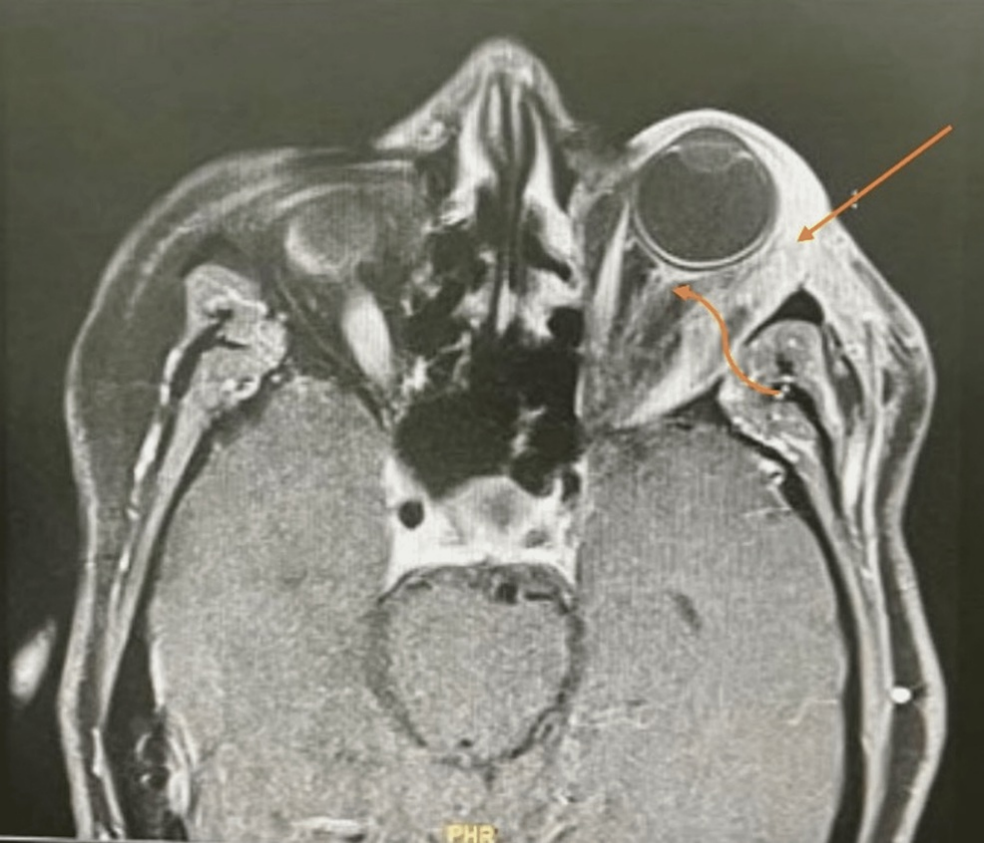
Postoperative MRI of the brain and orbit displays a residual left extraconal enhancing collection (straight arrow) This is associated with the progression of both preseptal and post-septal inflammatory changes as well as inflammation in the left optic nerve sheath (curved arrow), leading to secondary scleritis.
